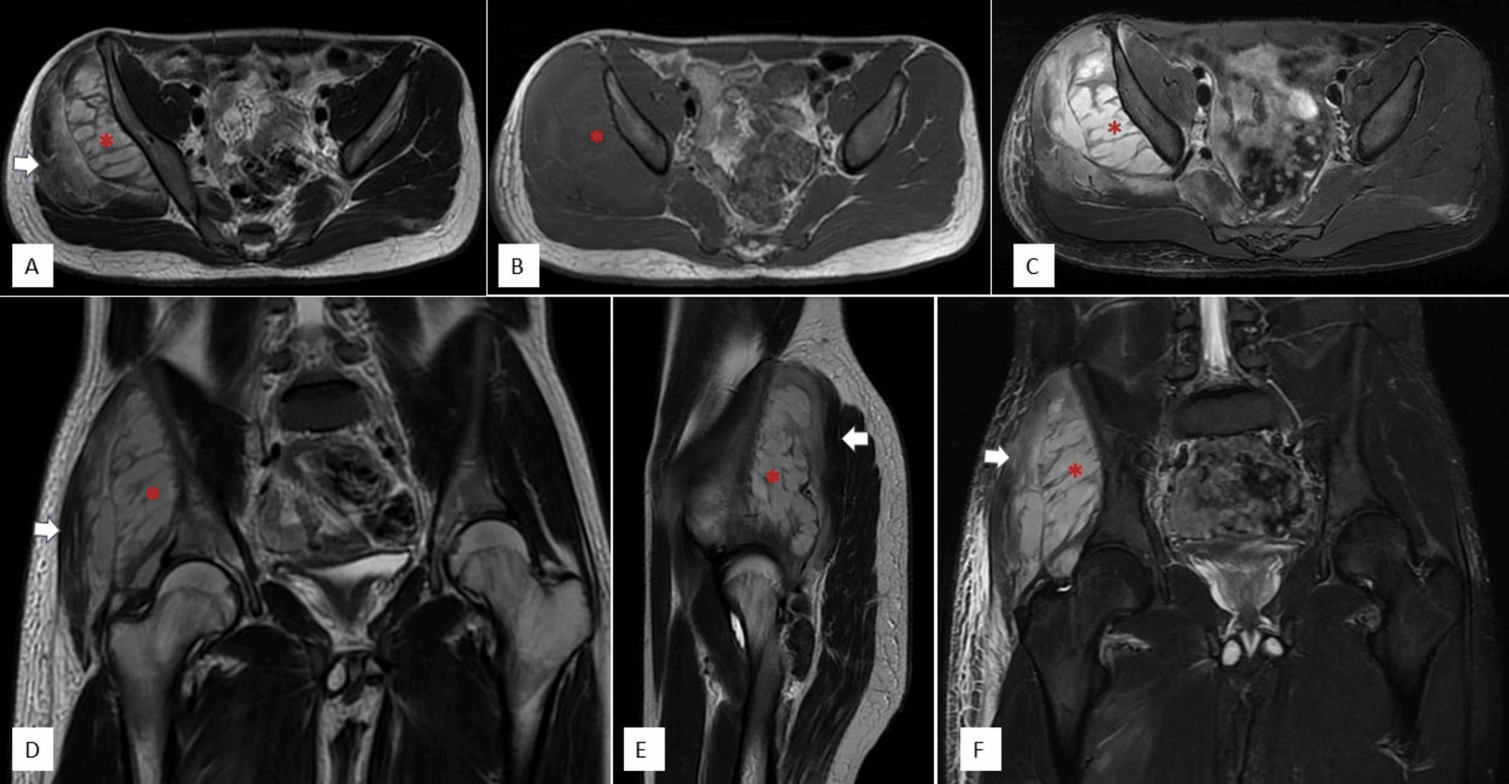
MRI of the pelvis demonstrating (A) axial T2, (B) axial T1, (C) axial T2 with fat suppression (water flex), (D) coronal T2, (E) sagittal T2, and (F) coronal short tau inversion recovery sequences. The right gluteus minimus muscle (red asterisk) appears bulky with extensive hyperintense signal on T2-weighted and short tau inversion recovery images, and iso- to hyperintense signal on T1-weighted images. Similar signal changes are noted in the adjacent gluteus medius (white arrow) and piriformis muscles, suggesting a myopathic or inflammatory process.
